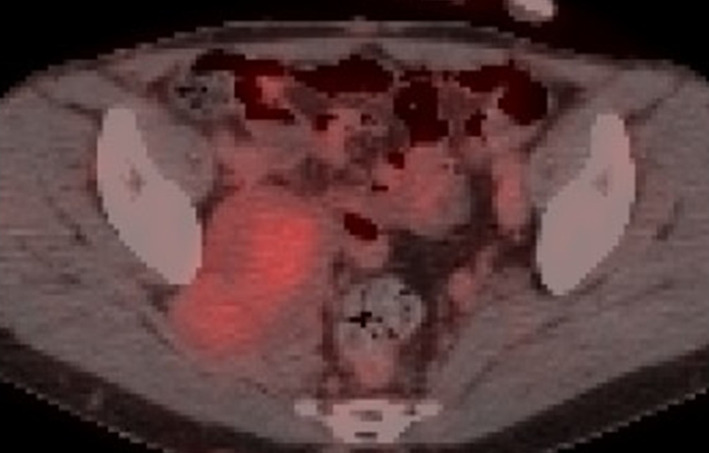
PET–CT. PET–CT shows high uptake (SUVmax 12.7) consistent with a pelvic mass
